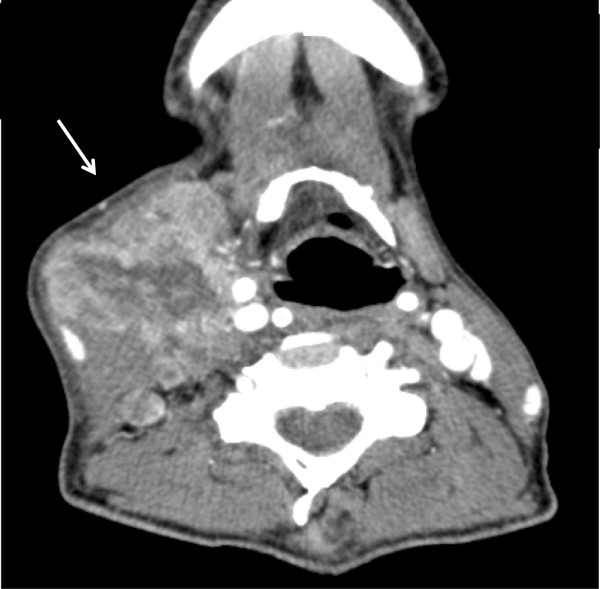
Computed tomography showed enlargement of lymph nodes on the right side of the neck (arrow).
